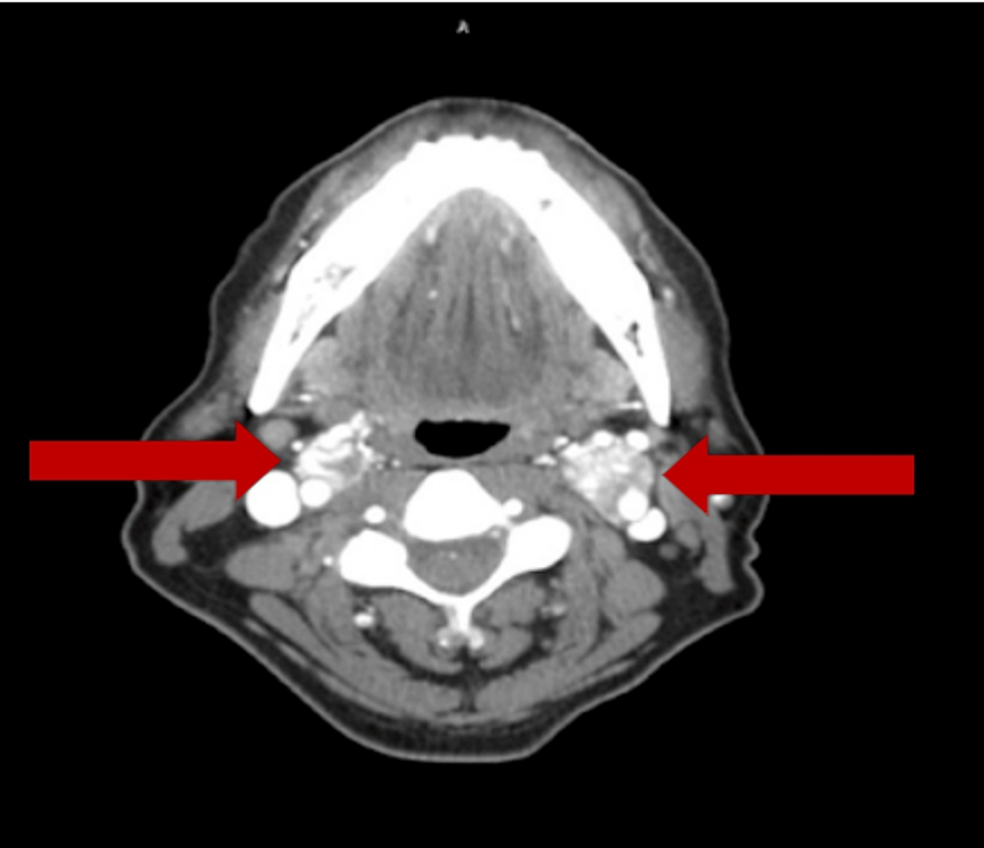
Axial CT angiogram showing bilateral heterogeneously enhancing carotid bifurcation masses (red arrows)
